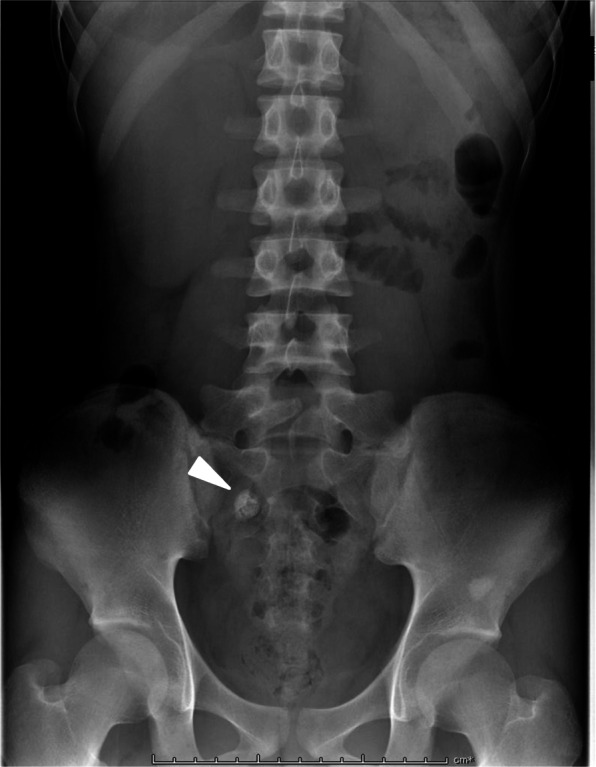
Preoperative abdominal radiography. A solitary stone with peripheral calcification and a lucent center was identified on abdominal radiography (arrowhead)
